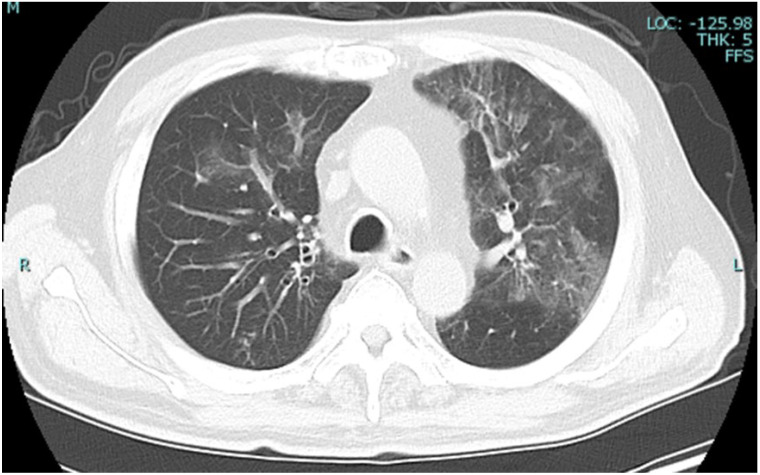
Chest CT on admission. Chest CT revealing non−segmental ground−glass opacities, predominantly in the upper lobes bilaterally. CT, computed tomography.
